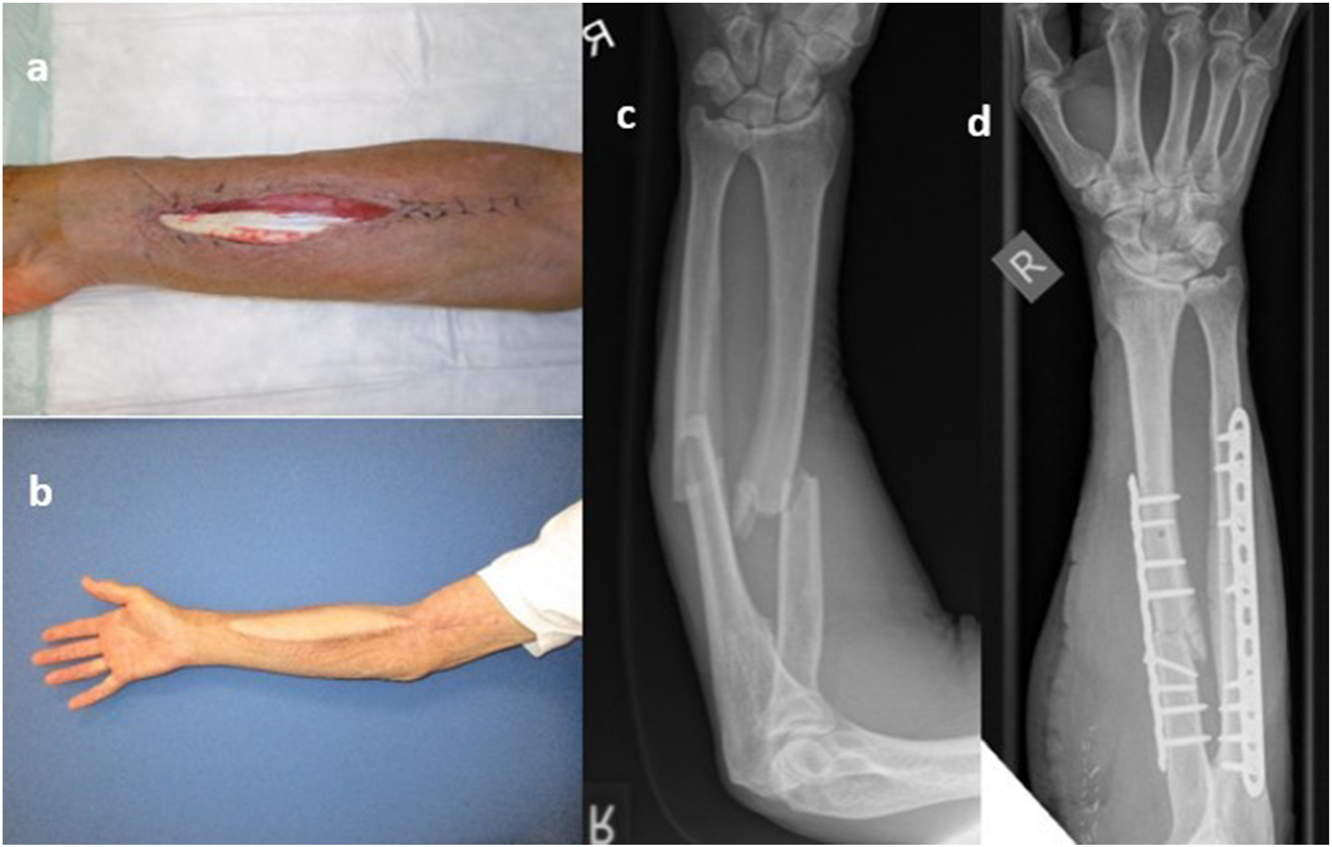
Male patient with soft tissue defect of the right forearm following open forearm fracture (a). (b) Two months postoperatively after defect coverage using a free anterolateral thigh (ALT) flap. (c)/(d) Pre- and postoperative X-rays of the right forearm.
